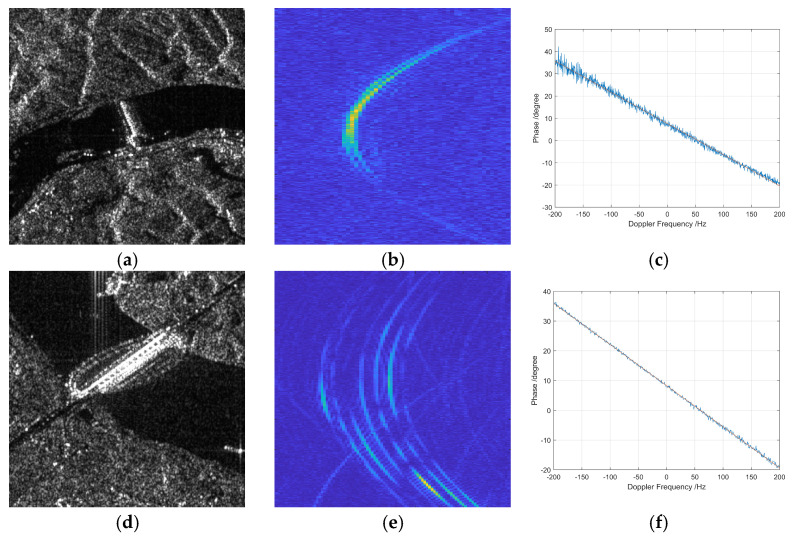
Multi-channel echo range migration curves of a man-made bridge target. (a,d) Image slice amplitudes; (b,e) Range migration curves after time-domain range compression; (c,f) Channel phase errors as functions of Doppler frequency.
